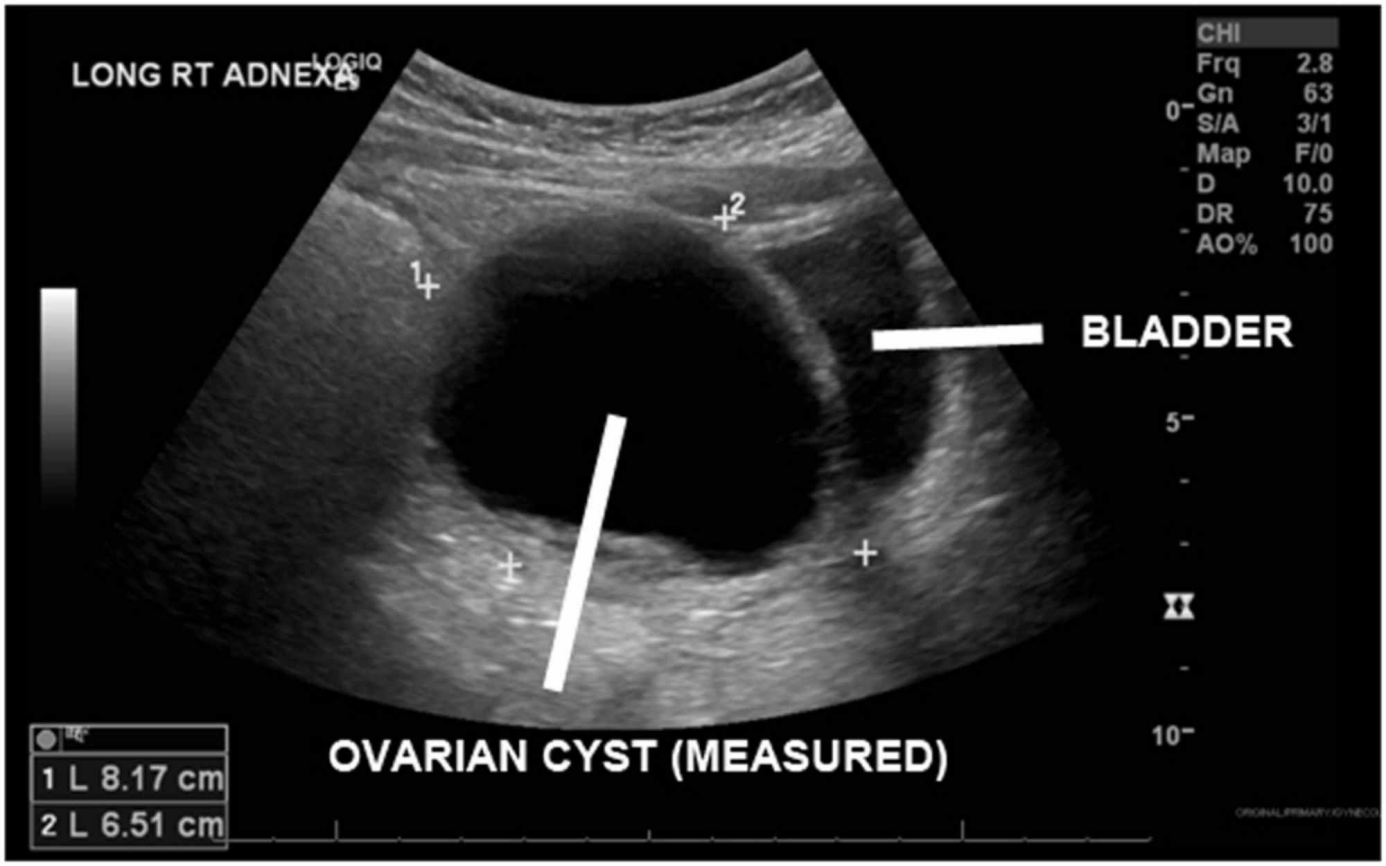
Trans-abdominal pelvic ultrasound (right adnexa)
